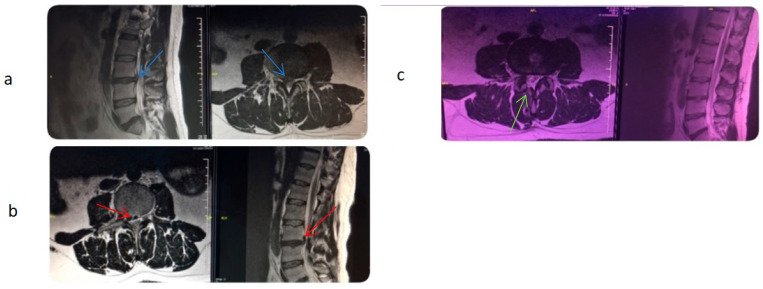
During the SELD procedure performed for a right paramedian disc herniation (blue arrow) at the L3-L4 level (a), the patient developed weakness in right knee extension following laser discectomy. Postoperative MRI findings (b) revealed cranial and foraminal migration of the paramedian disc (red arrow). The patient underwent a right L3-L4 laminectomy (green arrow) and discectomy one day after the procedure. Postoperative MRI findings following the surgery are shown in (c).
